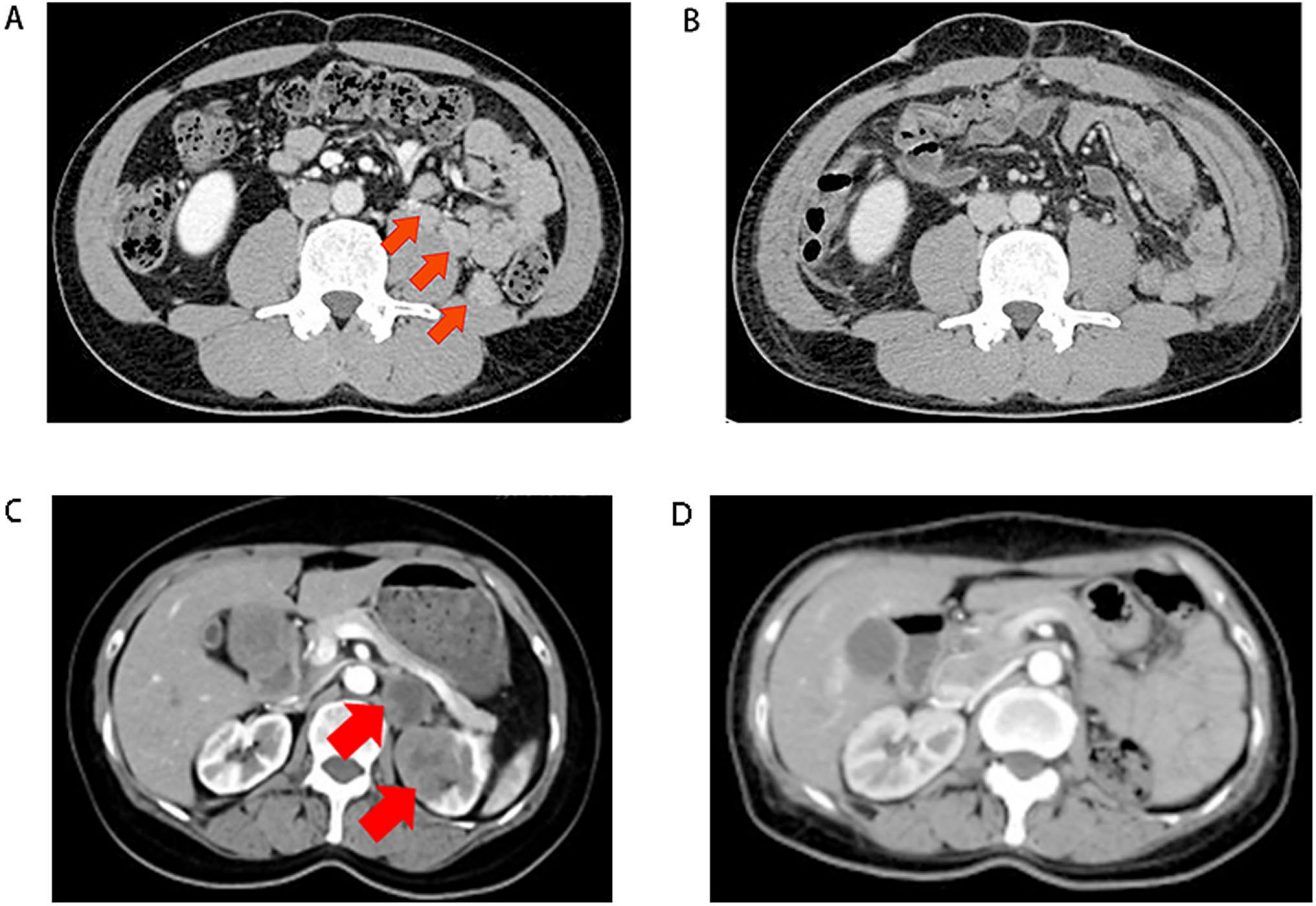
The CT scan of one of the patients underwent metastasectomy. A The preoperative CT scan of patients with metachronous metastasis who underwent metastasectomy. The arrow points to retroperitoneal metastasis lesions. B The postoperative CT scan of patients with metachronous metastasis who underwent metastasectomy. C The preoperative CT scan of patients with synchronous metastasis who underwent metastasectomy. The arrow points to retroperitoneal metastasis lesions. D The postoperative CT scan of patients with synchronous metastasis who underwent metastasectomy
